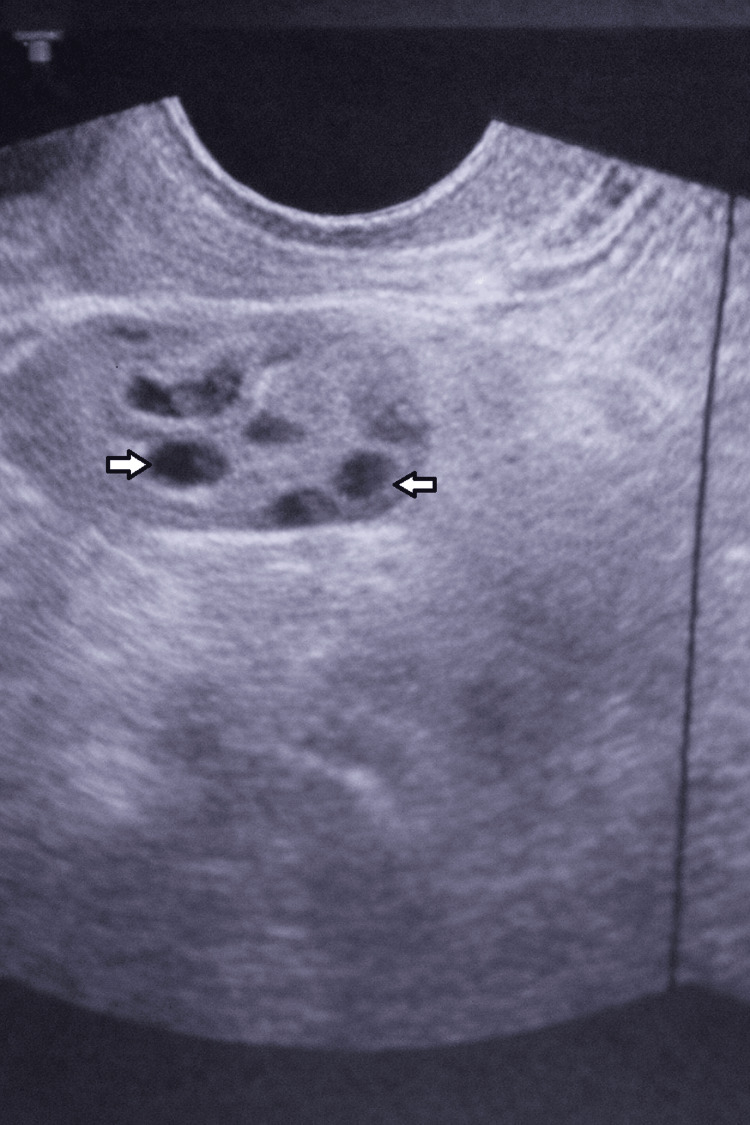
Abdominopelvic ultrasound showing multiple right adnexal masses of unknown origin
